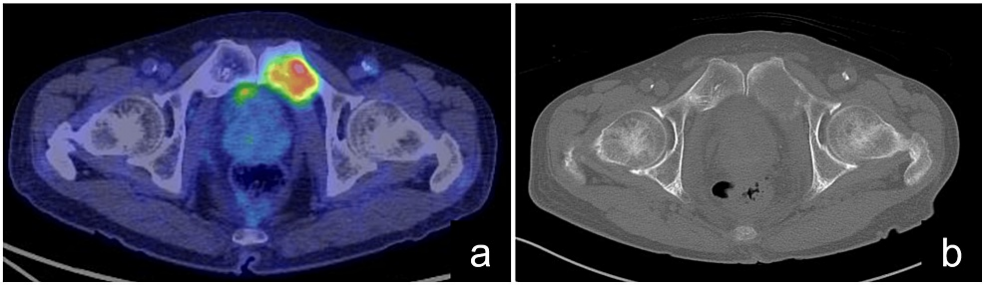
Metastatic pelvic tumor in the left pubic bone. (a) Positron emission tomography-computed tomography revealed anomalous accumulation in the left pubic bone. (b) Computed tomography imaging detected myocytic alterations in the left pubic bone.
